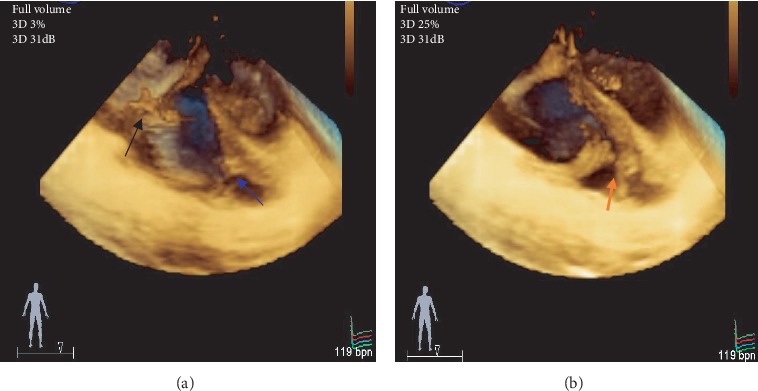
Three-dimensional transoesophageal views of the right ventricle showing the attachment point of the anterior papillary muscle ((a) blue and (b) orange arrows) and flail anterior tricuspid leaflet with ruptured papillary muscle ((a) black arrow).
